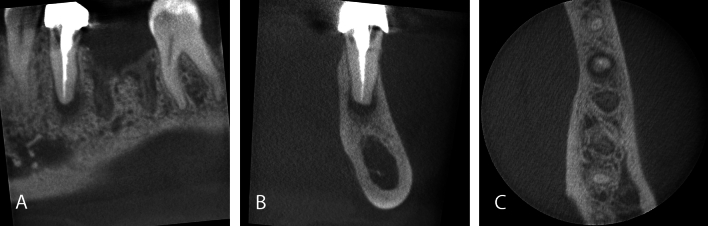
Small volume (4 × 4cm) CBCT scan of a 36-year-old male patient for implant pre-treatment examination in lower first molar position. A periapical radiolucency (apical lesion) of the root-treated neighbouring second premolar was identified as incidental finding. Sagittal (A), coronal (B) and axial (C) image
